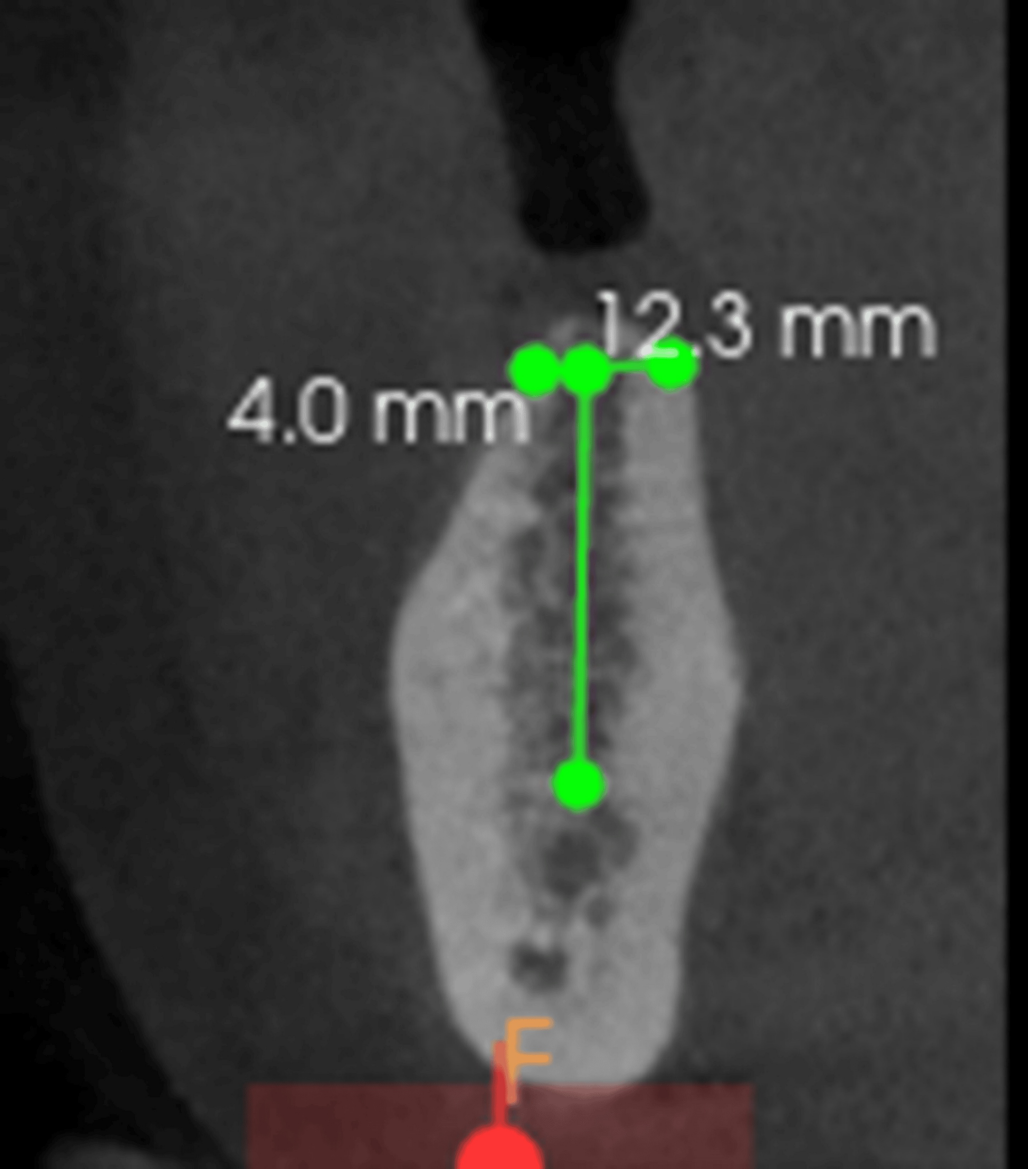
CBCT showing the width and height of the available bone CBCT, cone beam computed tomography
